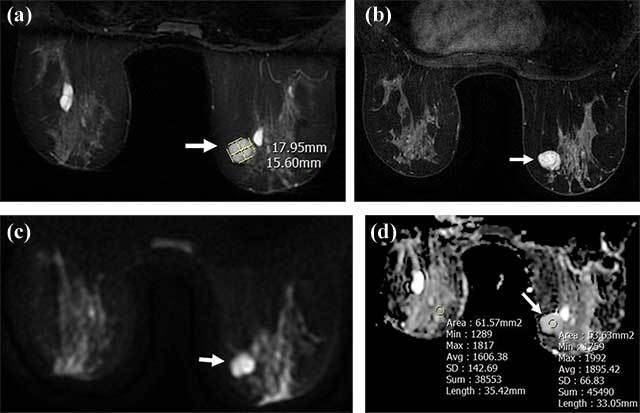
A well-circumscribed 18 × 15.6 mm mass lesion in the right breast upper-inner quadrant hyperintense (arrow) in T2-weighted images (a), homogeneously enhancing (arrow) in T1-weighted post-contrast images (b) without diffusion restriction in DWI (c, d); ADC and rADC are 1.895 and 1.179, respectively (d). Tru-cut biopsy results revealed fibroadenoma.
